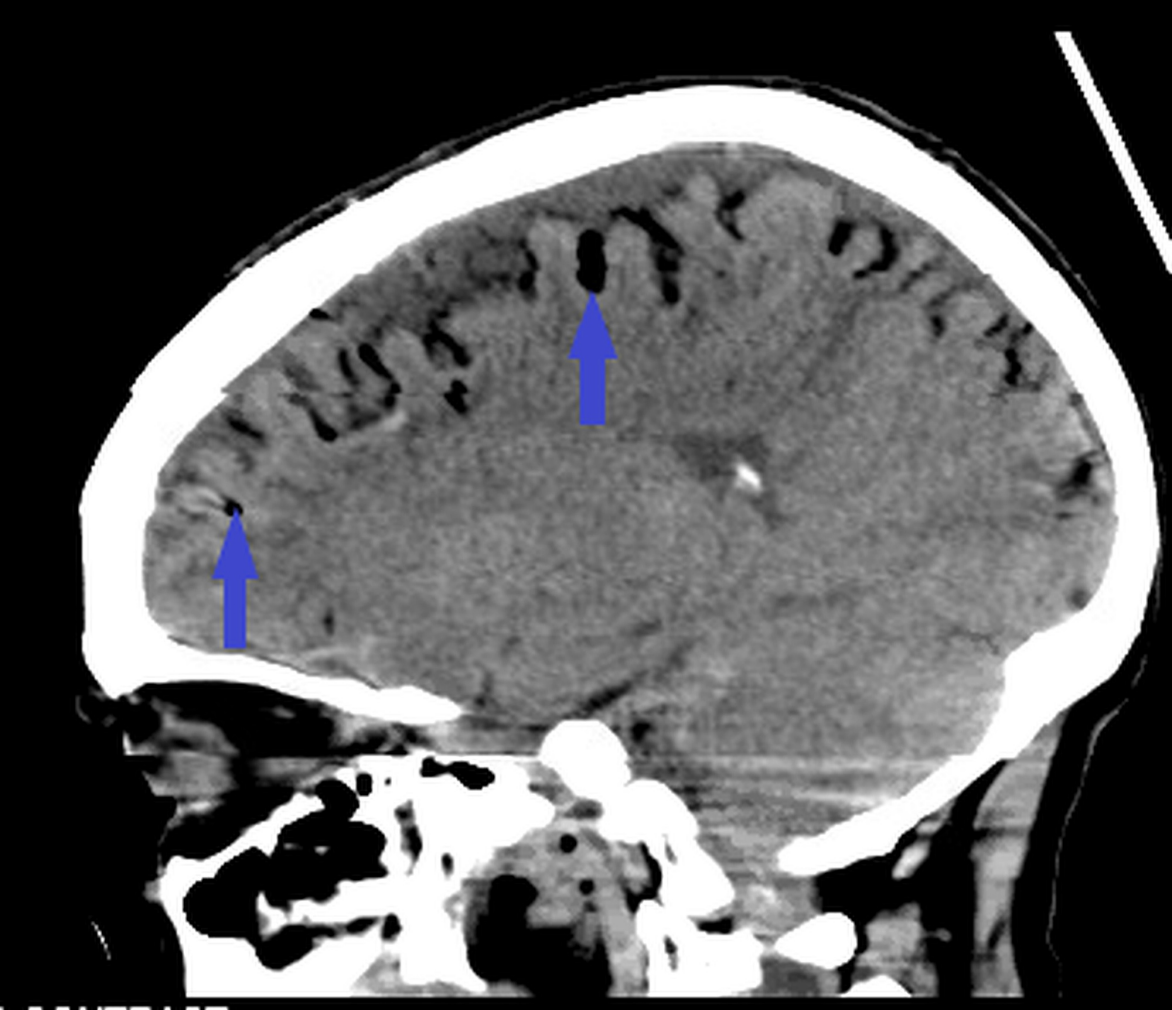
CT scan of the patient Note the presence of air bubbles (blue arrows) in the sulci diffusely (also known as the “air bubble” sign) CT: computed tomography
